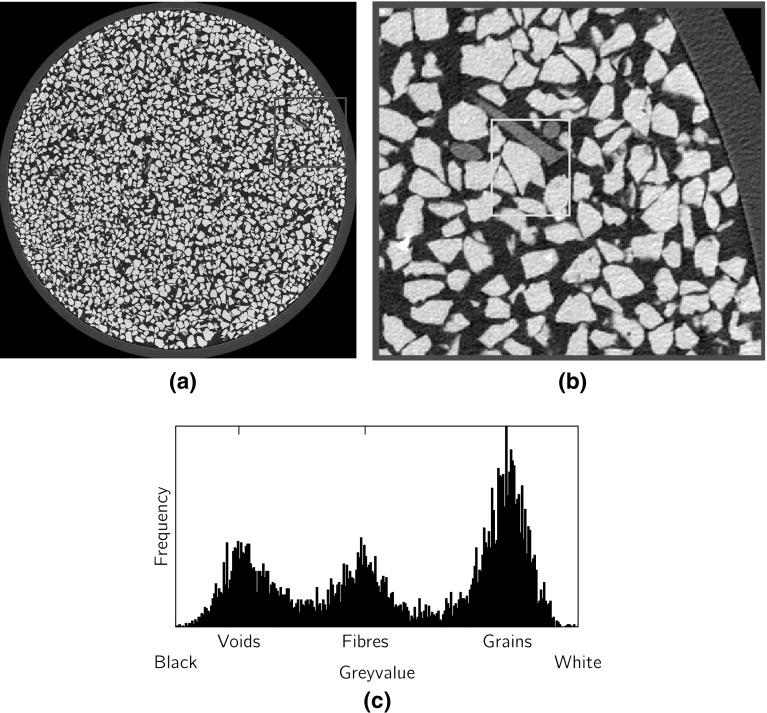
a Horizontal cross-section from an X-ray Tomography ($$12.5\,\upmu \hbox {m/px}$$) of a dry fibre-reinforced Hostun HN31 sand sample, b detail containing fibre phase, c histogram of the image intensity corresponding to the rectangle detail of (b)
